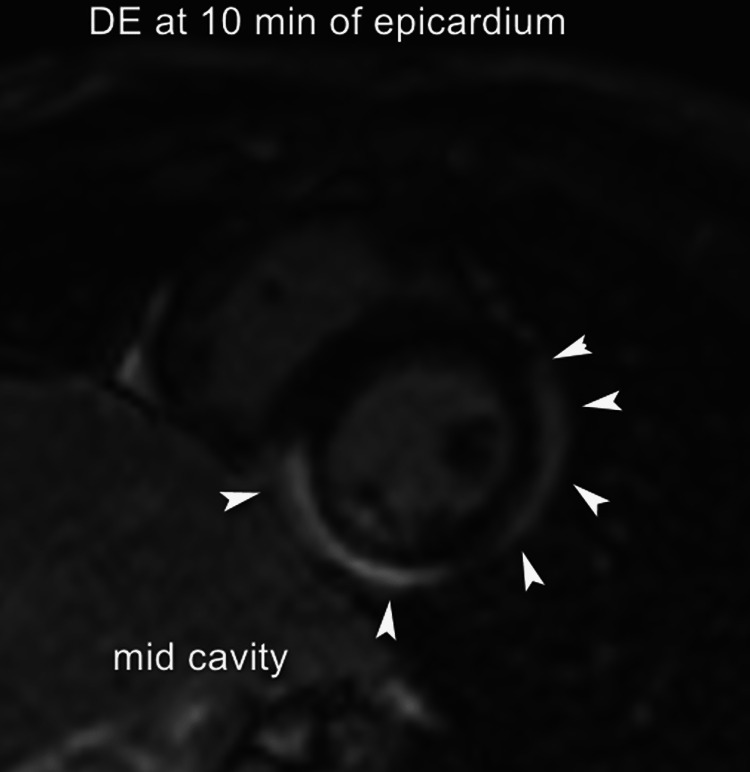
Cardiac MRI revealing mid-cavity inflammation
